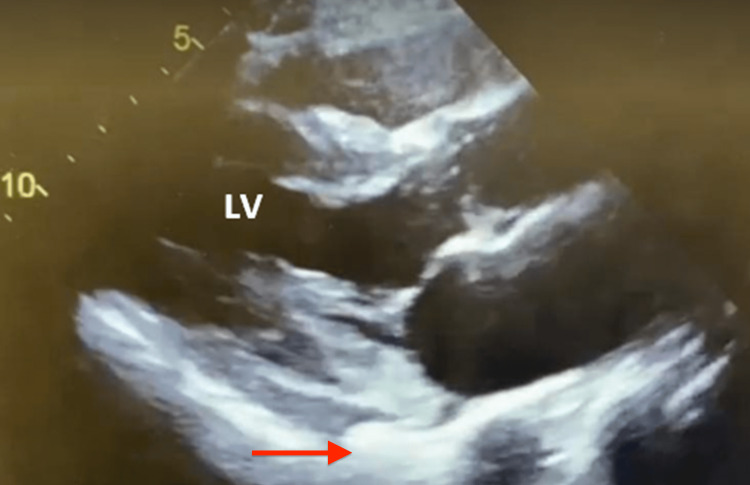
Echocardiogram three days after admission with LV EF 65%, no wall abnormalities, and no pericardial effusion (red arrow) LV EF: left ventricular ejection fraction
